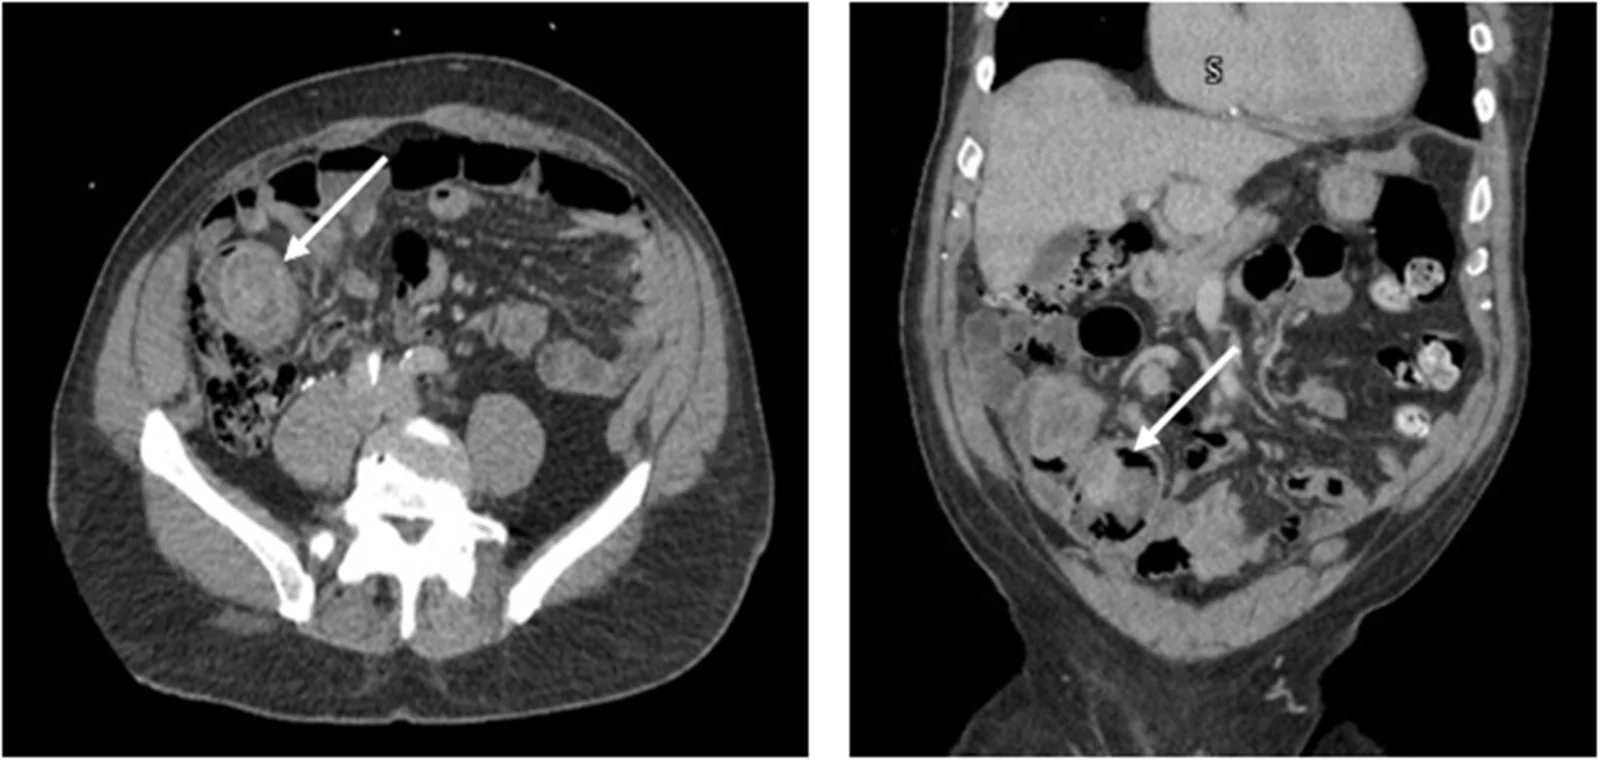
CT AP: Axial and coronal views showed a distal ileo-ileal intussusception (arrows) with associated wall thickening and hyperenhancement.
